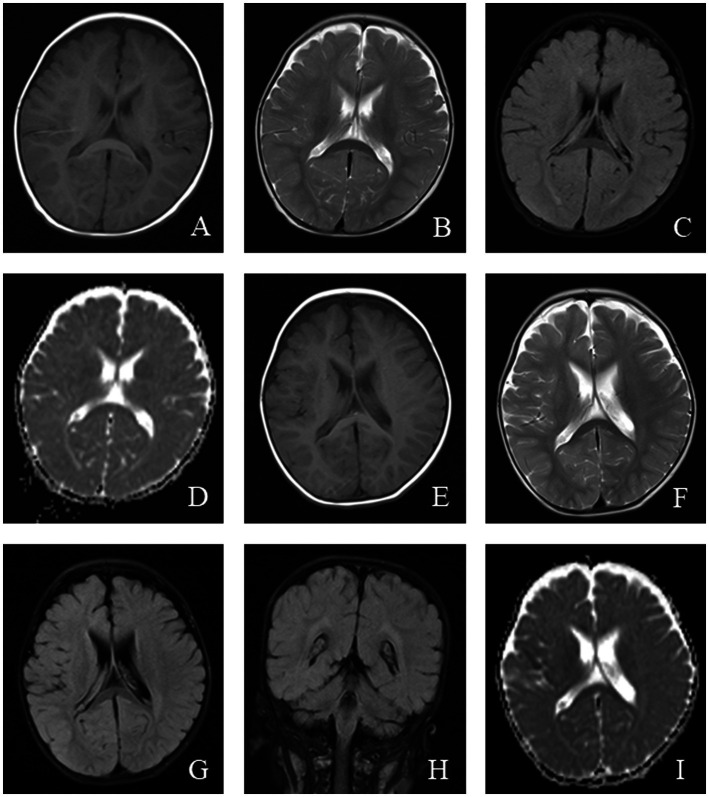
An 11-month-old male was diagnosed with Alpers syndrome due to persistent left limb convulsions for 4 h. Initial head MRI showed: (A–C) No abnormal signals in T1WI, T2WI, and dark-fluid images, small ventricles, and no displacement of midline structures in the brain tissue. (D) Bilateral symmetrical low signal intensity was observed on the ADC map of the caudate nucleus. Reexamination after 3 months: (E–H) Patchy abnormal signals were observed around the bilateral ventricles. T1WI showed iso-signal, while T2WI and fluid-attenuated inversion recovery (FLAIR) images showed slightly high signal intensity. (I) Bilateral symmetrical low signal intensity was still visible on the ADC images of the caudate nucleus.
